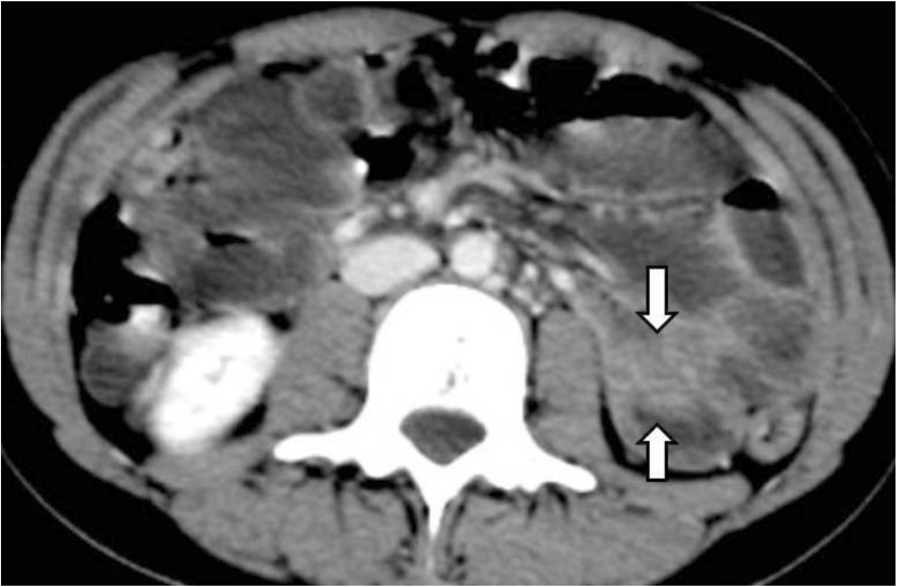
Computed tomographic enteroclysis. Neutral enteral contrast shows a polypoid intraluminal mass with remarkable enhancement (arrows).
